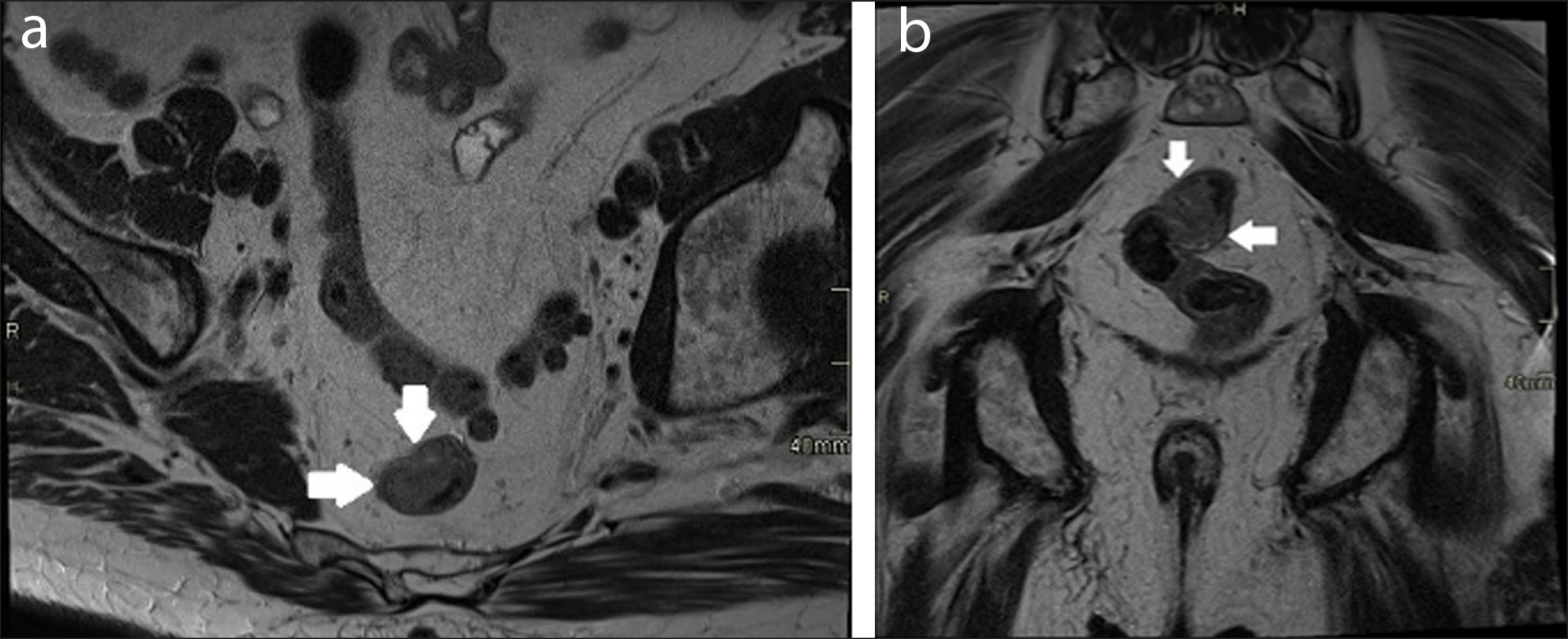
Axial (a) and coronal (b) T2-weighted sequence of the lower pelvis, showing a iso-intense semi-circular, sessile mass in the middle 1/3 of the rectum (white arrows).
